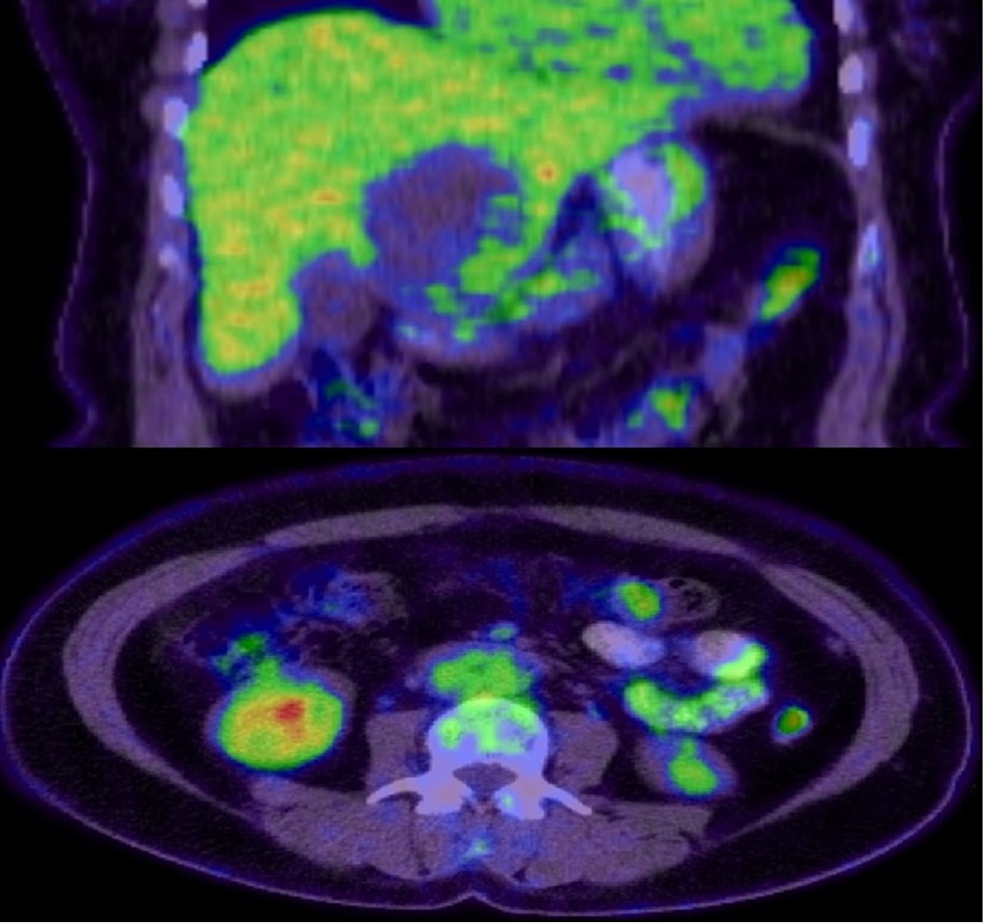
PET-CT showing mild radiotracer uptake by the left hepatic lobe complex cystic lesion (SUVmax 3.8) and the peritoneal nodule in the left side of the abdomen PET-CT: positron emission tomography-computed tomography
